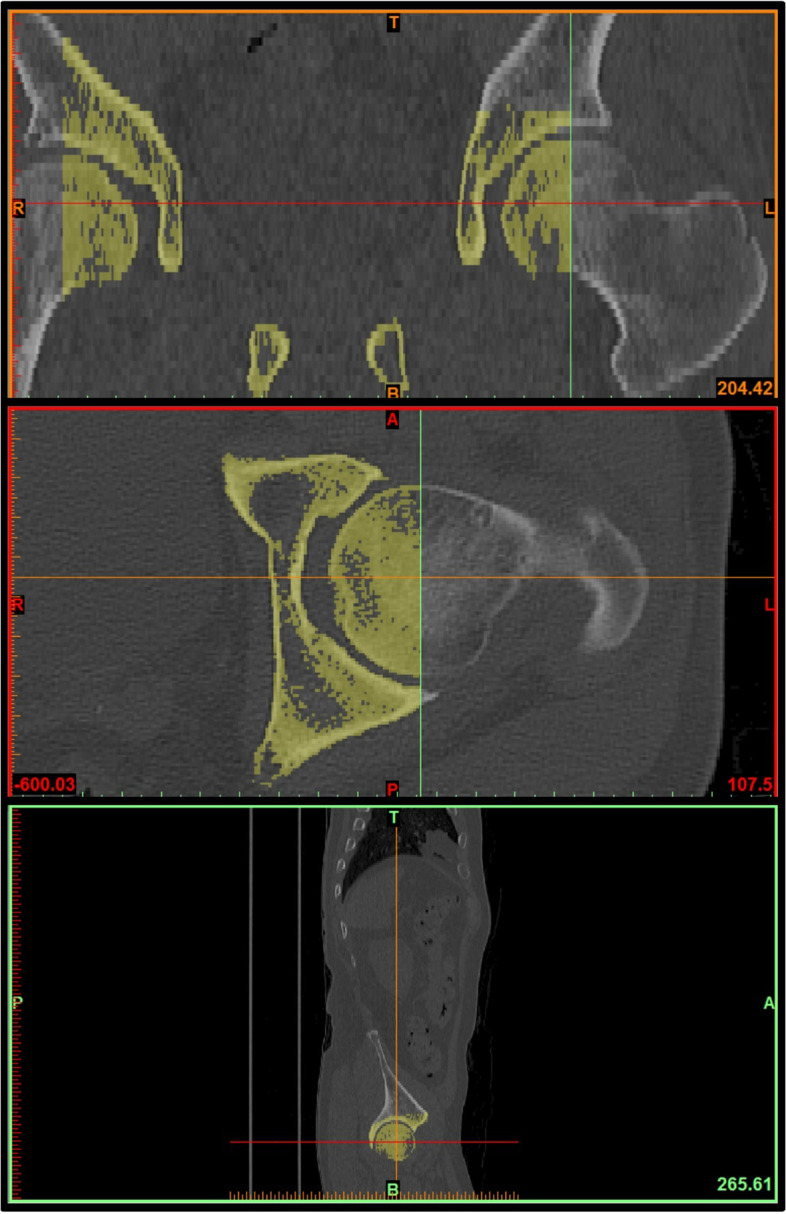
3D reconstruction of femoral head for pelvic incidence; Acquired CT images were performed and modified 3D reconstruction images using 3-Matic program. Segmentation of both femoral heads into halves based on the coronal, sagittal, and axial plane
